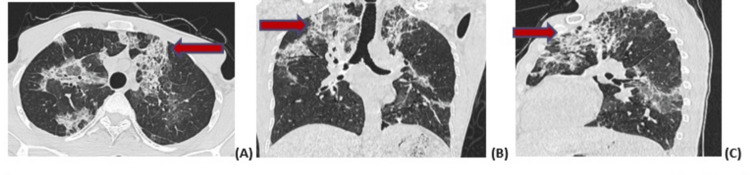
Figure 1 (A-C): CT scans in axial, coronal, and sagittal sections showing crazy paving (red arrow) extending to both lung fields, which can be indicative of eosinophilic lung disease among other conditions
